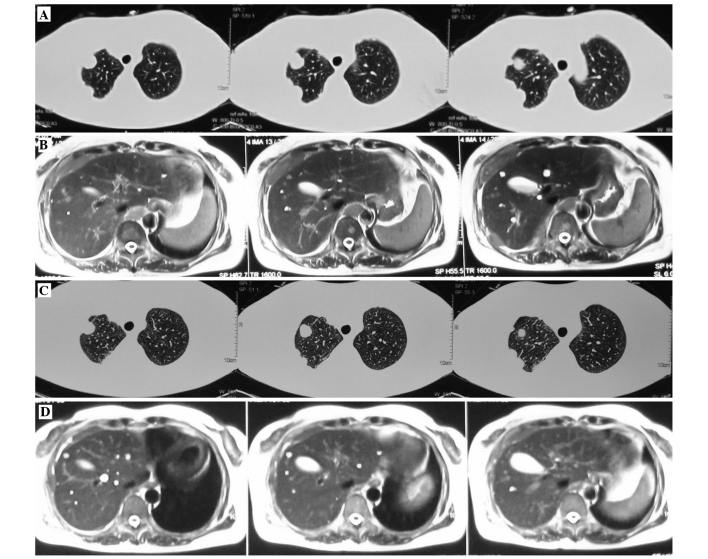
(A) Thoracic computed tomography (CT) images prior to nab-paclitaxel chemotherapy. (B) Abdominal magnetic resonance imaging (MRI) revealing the presence of peritoneal metastasis prior to nab-paclitaxel chemotherapy. (C) Thoracic CT revealing that the metastatic lesion in the lung was reduced in size following nab-paclitaxel chemotherapy. (D) Abdominal MRI revealing that the metastatic lesion in the peritoneum was significantly reduced following nab-paclitaxel chemotherapy.
